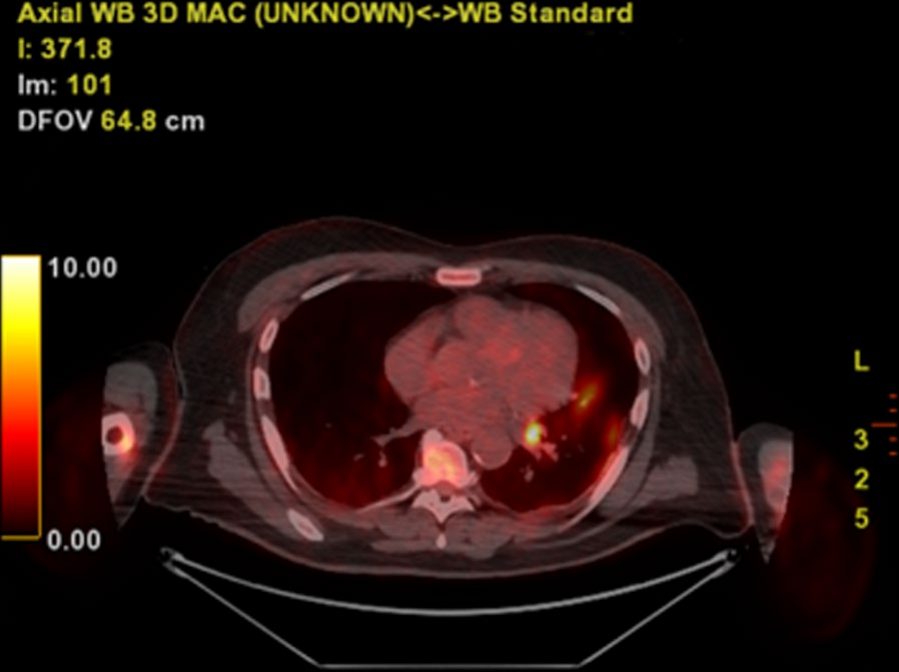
PET-CT scan showing no FDG uptake at the aortic valve region
